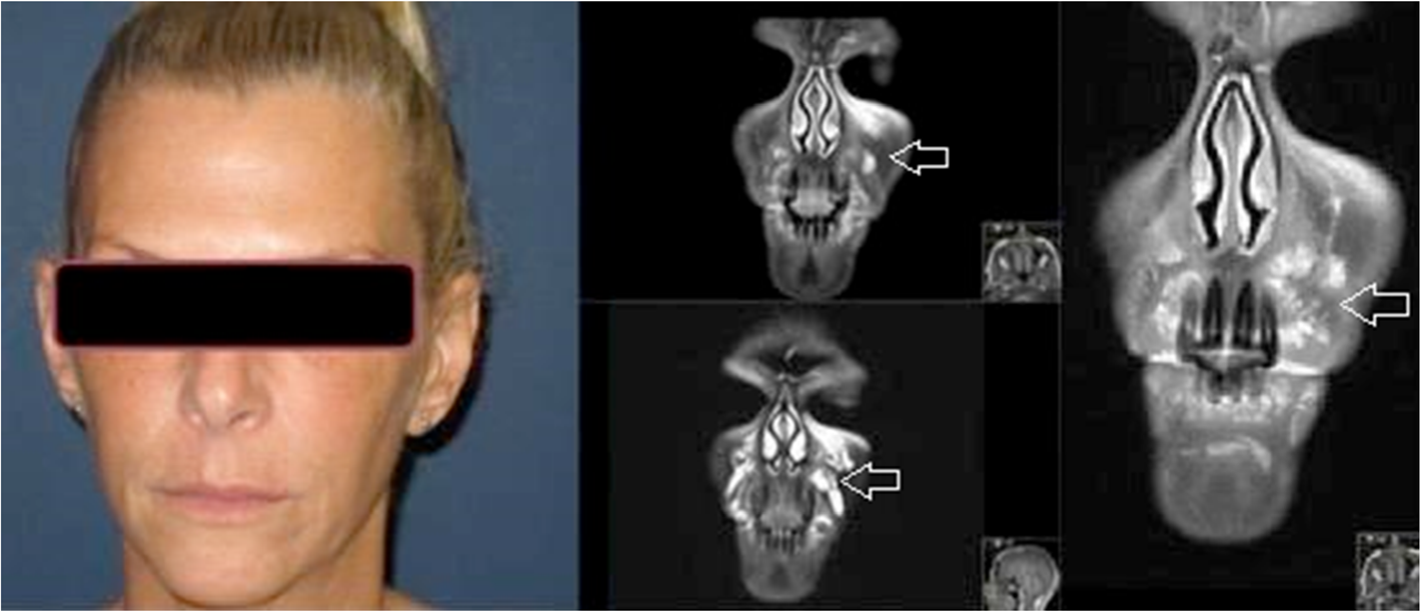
Delayed reaction to calcium hydroxyapetite, coronal MRI (T1FS, T2FS, T1FS with gadolinium). Note the filler in the left infraorbital triangle (*open arrows*), with the delayed reaction extending to the nasolabial sulcus, causing the gross asymetry
